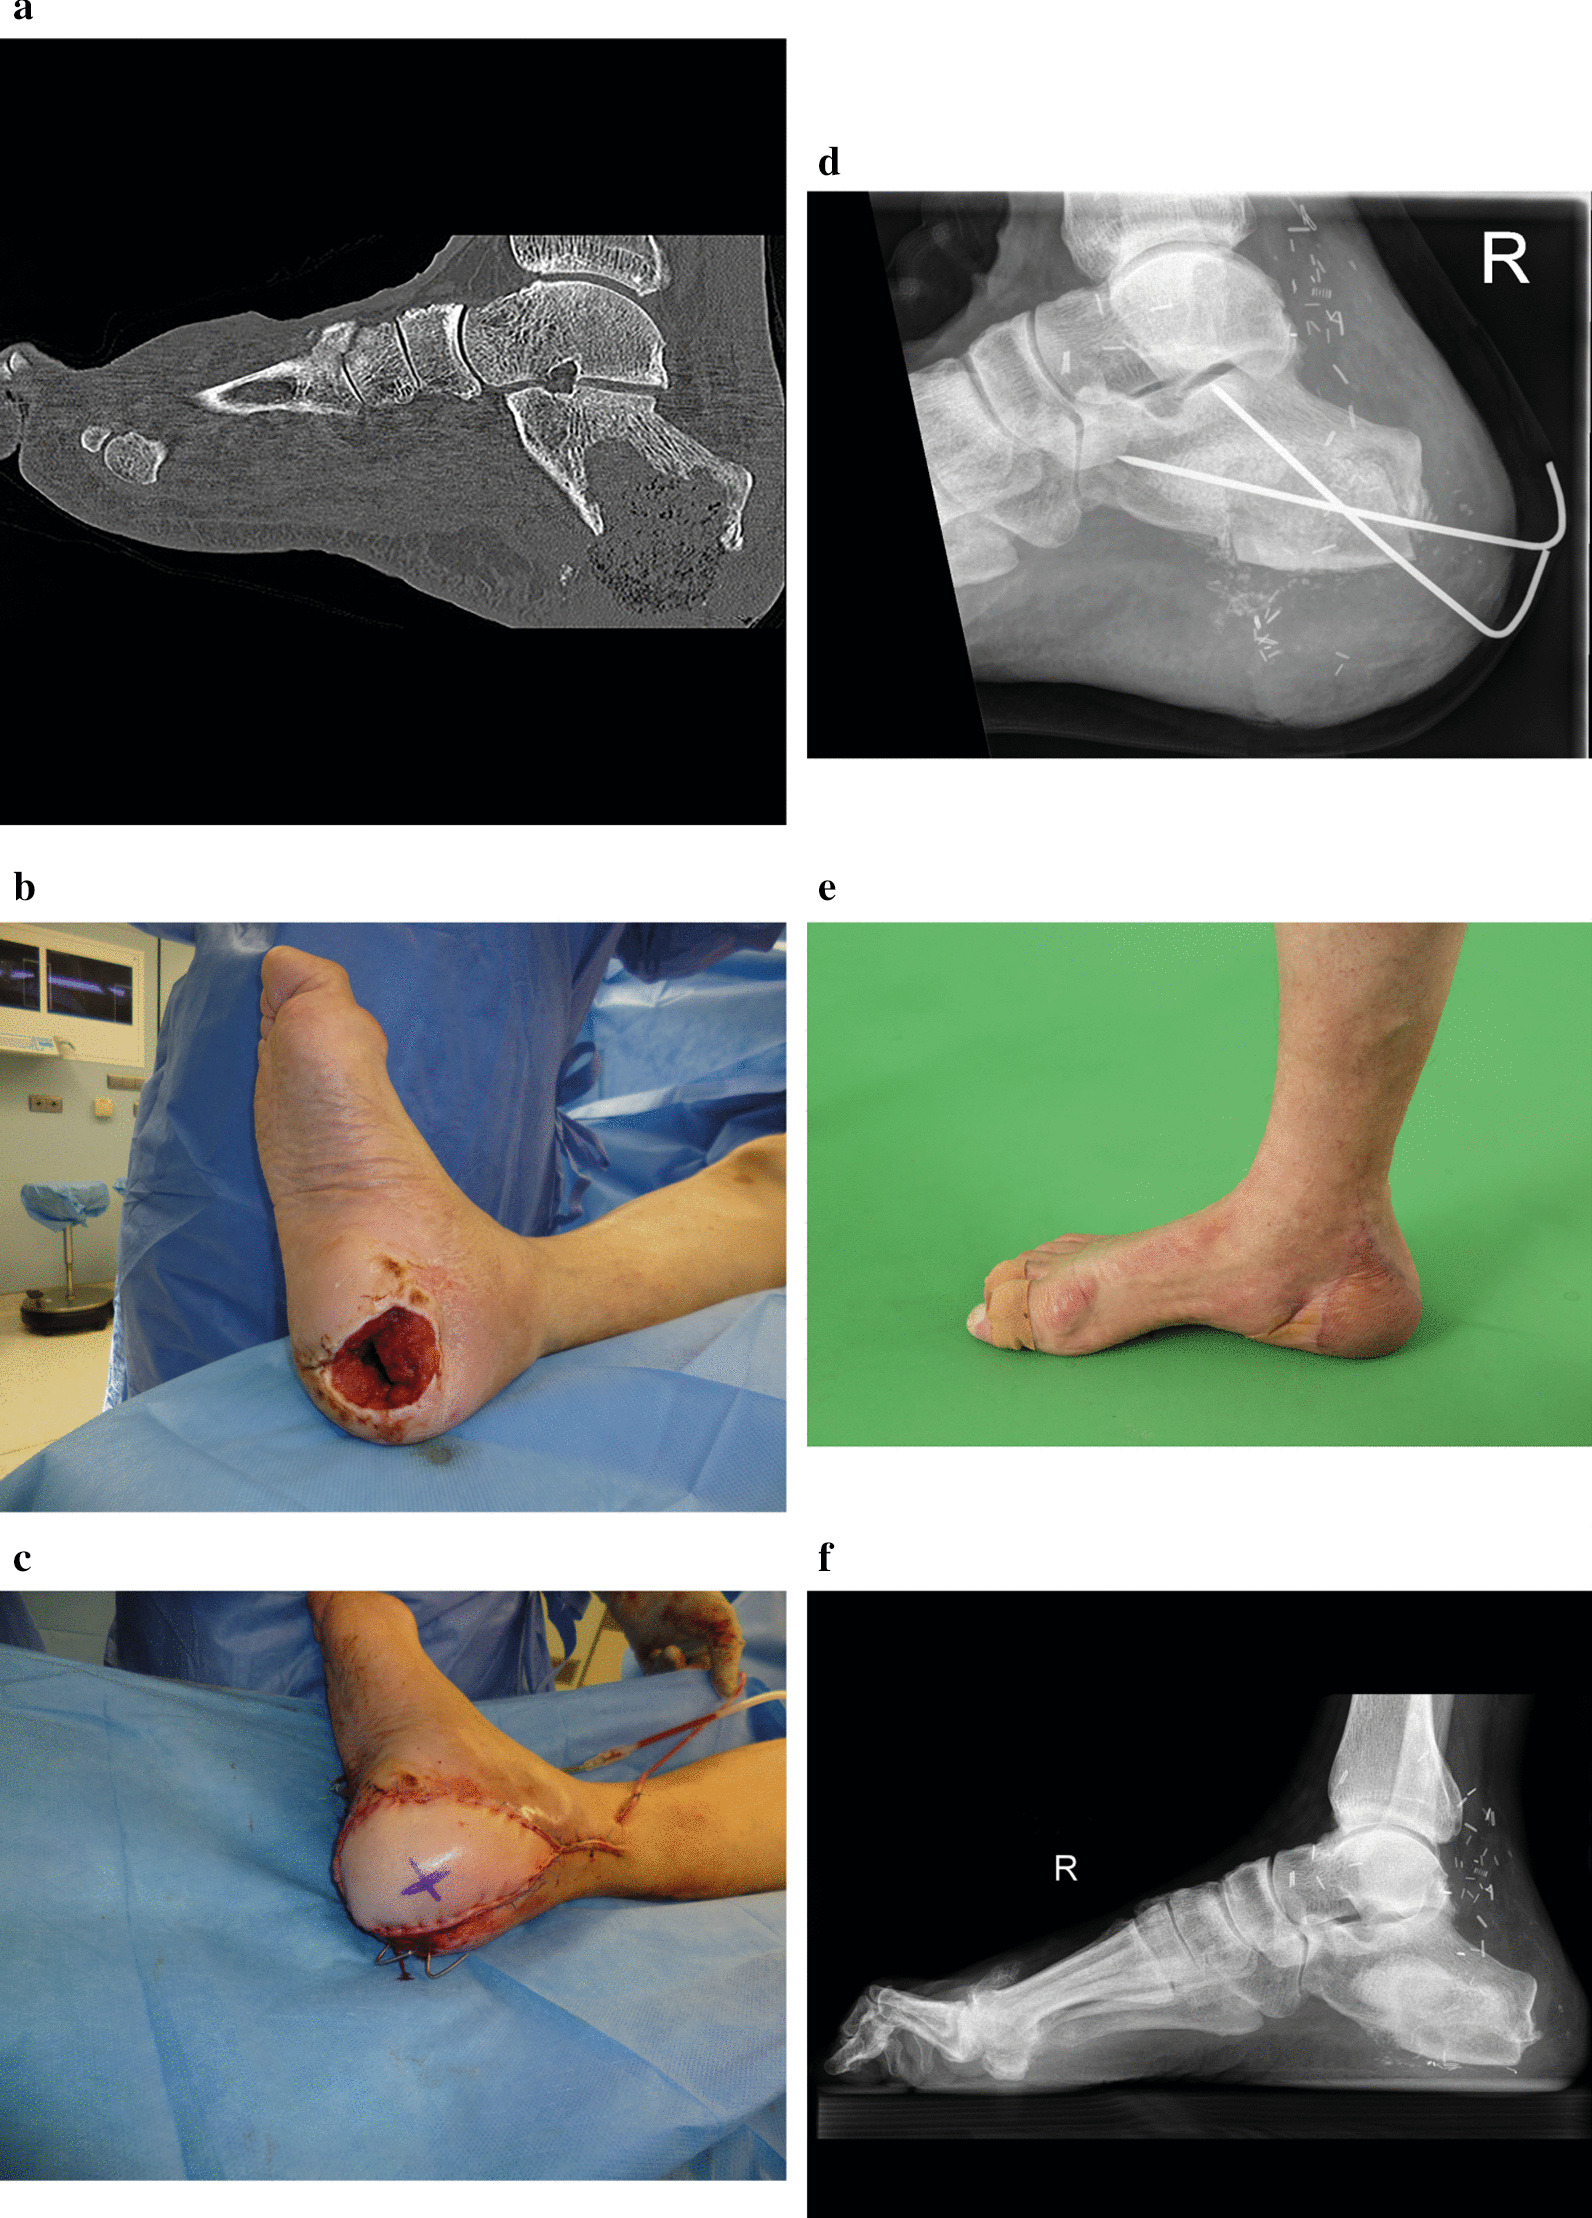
Limb-threatening chronic osteomyelitis of the calcaneus with subtotal resorption of the calcaneus and concomitant heel ulcers in a 66-year-old male patient. a Computed tomographic imaging of the right foot with subtotal bone resorption of the calcaneus due to chronic osteomyelitis. b Intraoperative lateral view of the right lower leg and foot with a major soft-tissue defect in the right weight-bearing heel before reconstruction. c Postoperative lateral view of the reconstructed heel. The bone defect has been reconstructed with a free vascularized iliac crest flap and the soft-tissue defect with a free groin flap. In-flap anastomoses were performed between the nutrient vessels of the iliac crest and groin flap and further anastomoses performed to the A. tibialis posterior. Furthermore, 15 cc bioactive glass scaffold implantation was performed. d X-ray of the right foot 21 days after internal fixation of the iliac crest bone segment by two k-wires. e + f Longterm follow-up after 1 year showing full weight-bearing and sufficient consolidated right calcaneus in the X-ray
